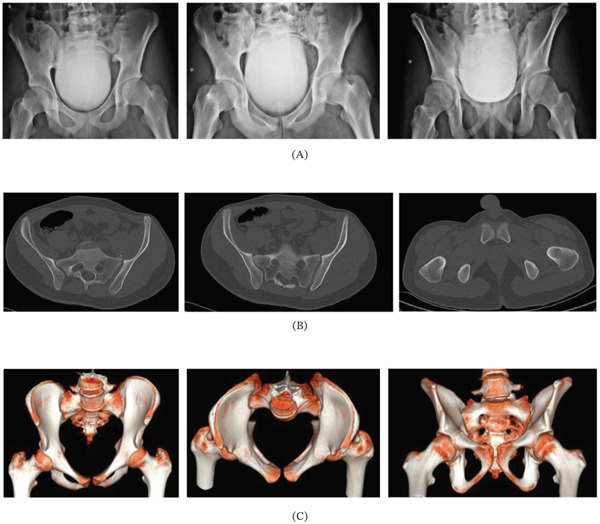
Preoperative imaging. (A) Preoperative pelvic radiographs in the anteroposterior (AP), inlet, and outlet views. (B) Axial CT scan images demonstrating the first and second sacral segments and the pubic symphysis. (C) Three‐dimensional CT reconstruction of the pelvis.
